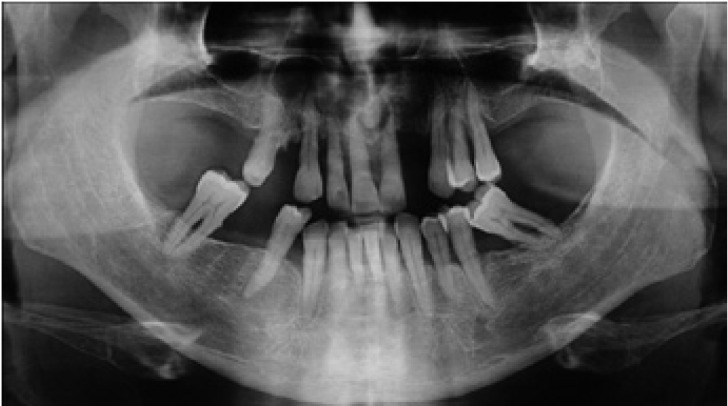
Panoramic radiograph of a KCOT occupying the right maxilla, between the right second incisor and the first premolar teeth. The border of the lesion is not readily apparent.
